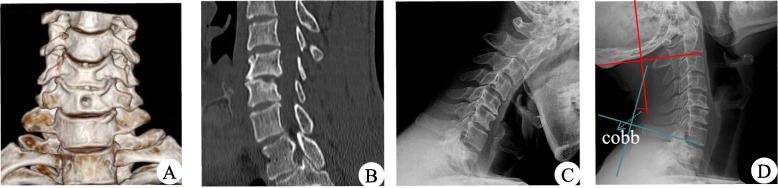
One year after the operation, CT scanning showed that the bony passage healed well and X-ray indicated that the physiological curvature of the cervical spine remained intact, with no cervical instability
